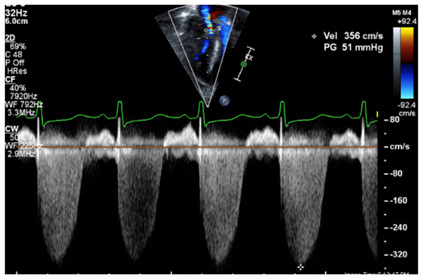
Legend: The tricuspid regurgitant jet velocity provides information on the velocity of flow during systole from the RV to the RA. This allows to estimate, using the Bernoulli equation, the pressure gradient between the RV and the RA. A full envelope is generated when the line of interrogation is parallel to the tricuspid regurgitant jet. With an estimated RA pressure (typically 0–5 mmHg in a normal setting, but likely further increased in RV diastolic impairment), one is able to estimate the peak systolic RV pressure. Assuming that the RV and pulmonary arterial pressure are equalized at the peak of systole, one may infer the peak systolic pulmonary arterial pressure. The velocity measurement should be performed along the contour of the spectral Doppler envelope, avoiding overestimation of the measurement. In this case, the peak systolic RV–RA gradient has a velocity of 3.56 m/s, providing an estimated RV–RA pressure gradient of 51 mmHg (4 × 3.56^2^). With an RA pressure of 5 mmHg, this provides a peak systolic RV pressure estimated at 56 mmHg (abnormal > 40 mmHg).
